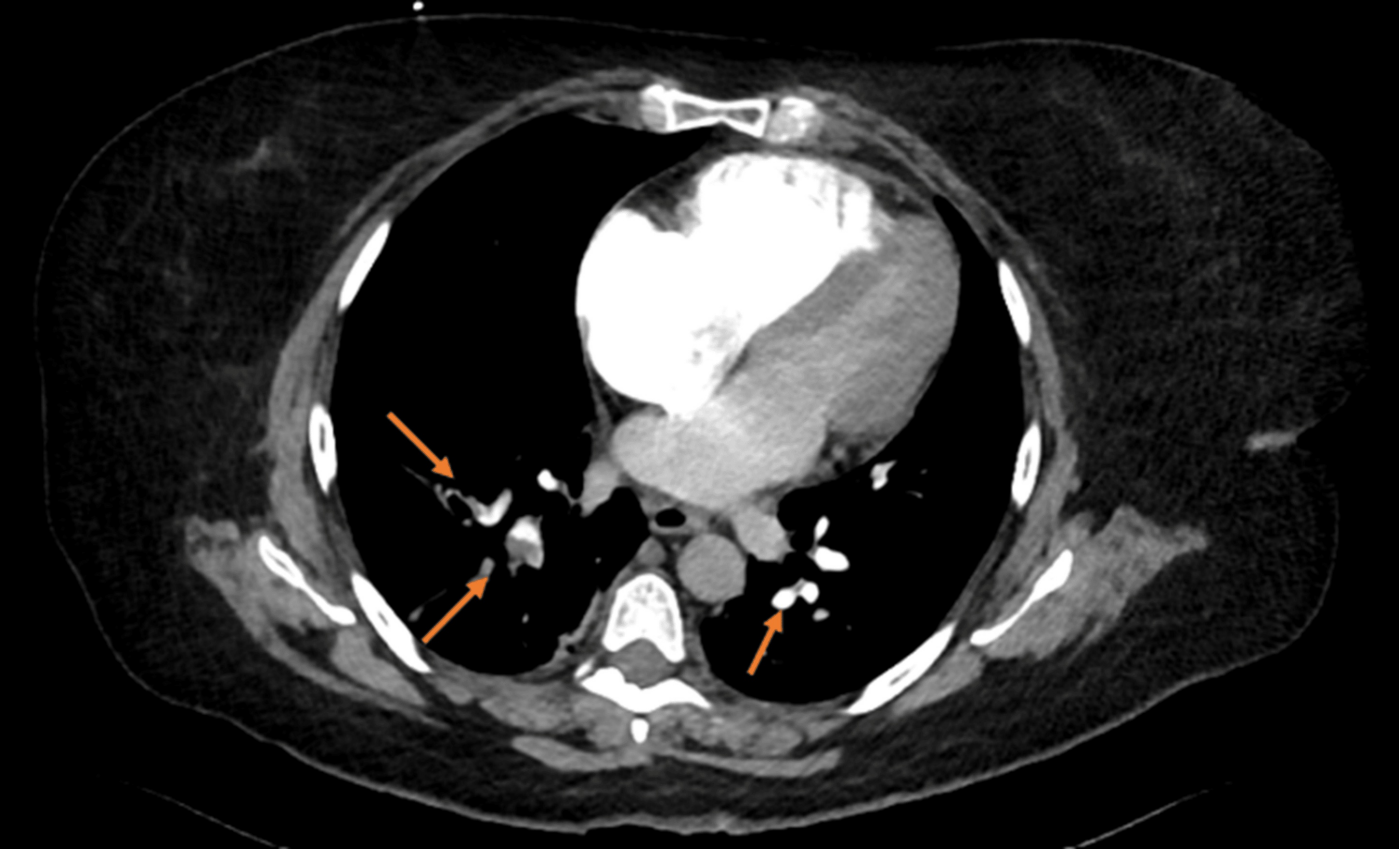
CTPA with orange arrows indicating multiple segmental and subsegmental pulmonary artery thrombosis CTPA: computed tomography pulmonary angiography
